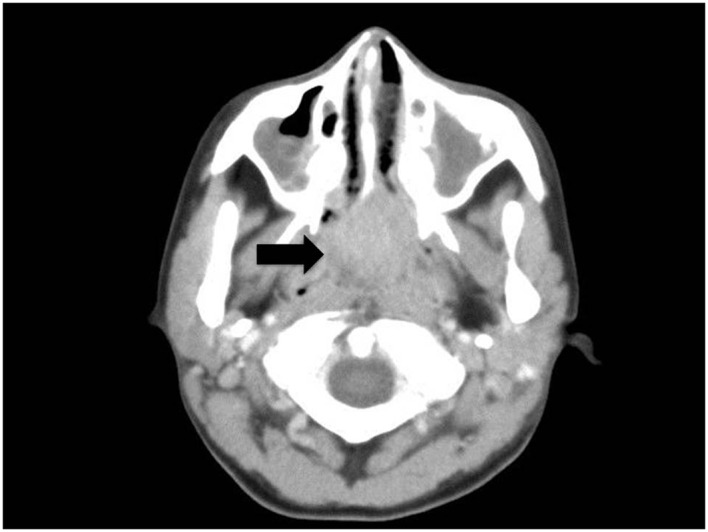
CT revealing a mass (arrow) occupying almost the entire nasopharyngeal space. CT, computed tomography.
